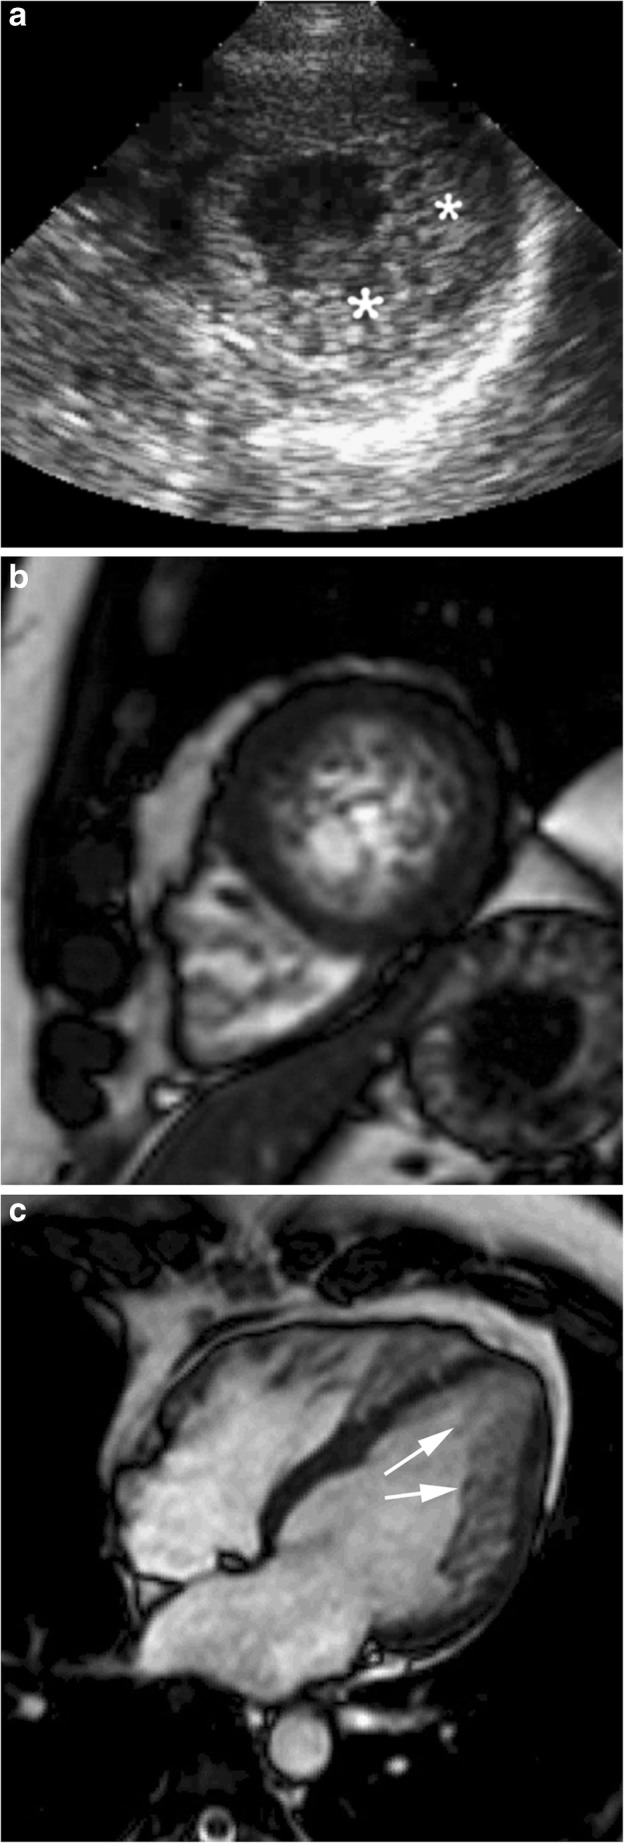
A 45-year-old woman with reduced ejection fraction and isolated LV non-compaction. a Echocardiographic findings were suggestive of apical hypertrophic cardiomyopathy (*asterisks*). b Short-axis cine SSFP MR image at end-diastole shows left ventricular apical trabeculated myocardium and thin epicardial compacted layer. End-diastolic ratio between non-compacted and compacted layers is greater than 2.3. c End-diastolic long-axis view SSFP image demonstrates the myocardial trabeculations (*arrows*) and clearly depicts their extension from apex to lateral wall
